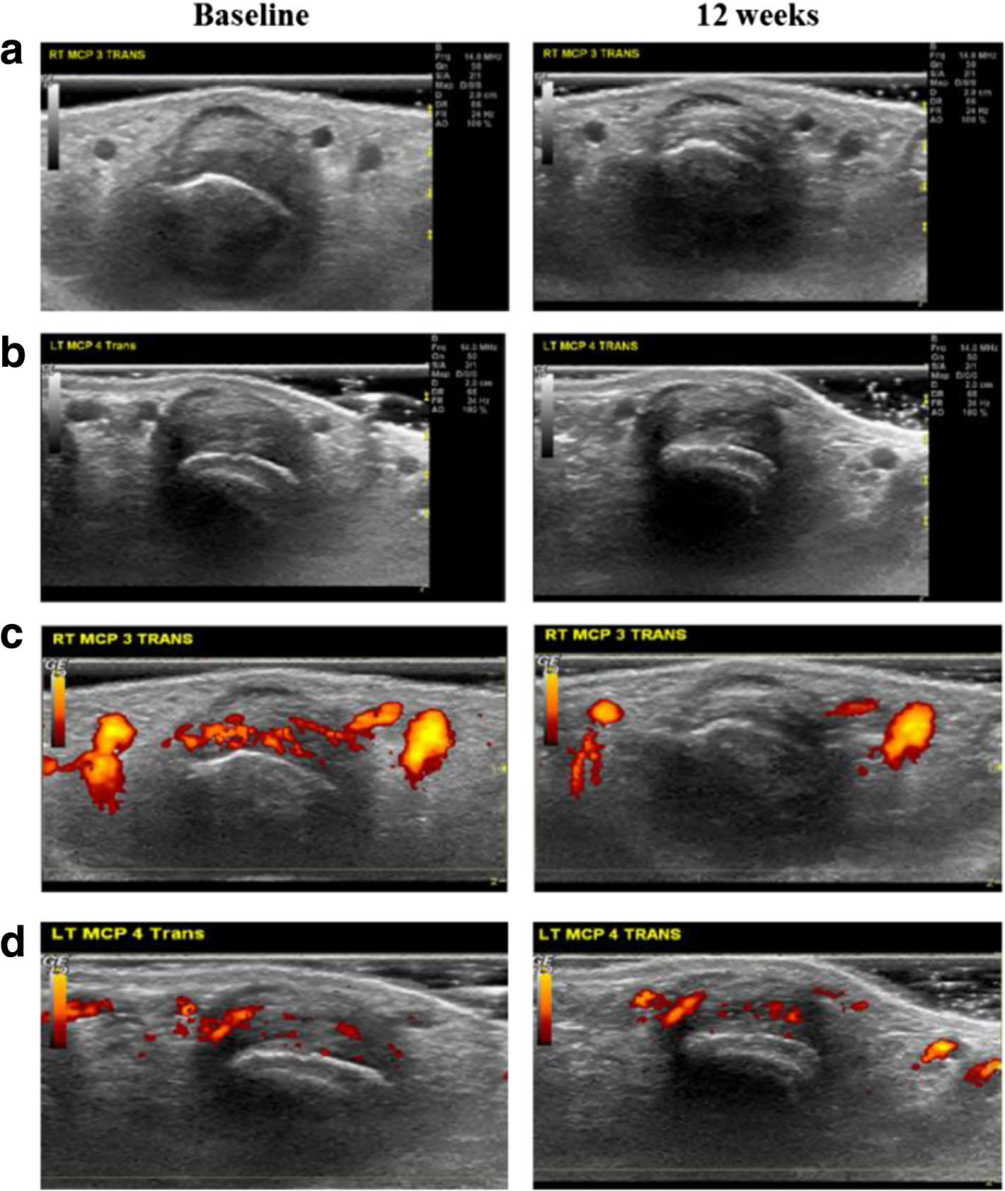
Differences between anti-TNF responders and non-responders in improvement in synovial thickening and vascularity. Representative ultrasound images of changes in synovial thickening (a and b) and synovial vascularity (c and d) in a metacarpophalangeal joint of a good responder (a and c) and poor responder (b and d) to anti-TNF therapy
